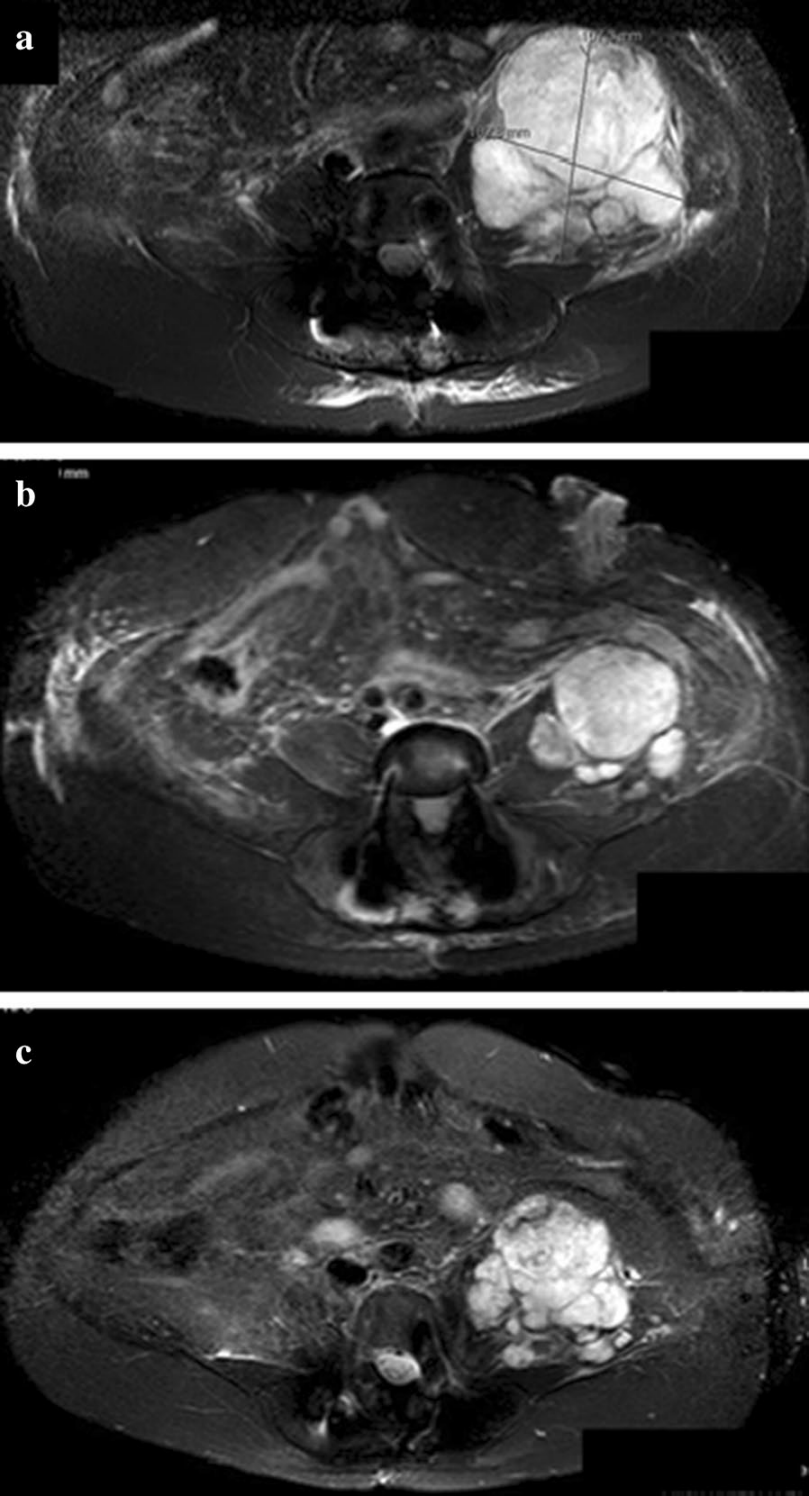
MRI images (T2 gadolinium and fat suppression) of patient 5 treated with sunitinib. a MRI scan at start of sunitinib showing a large retroperitoneal metastasis of a primary lumbar spine chordoma. Measurements: 10.3 × 10.7 × 9.4 cm (LR × AP × CC). b MRI scan after 6 months of sunitinib showing a partial response according to RECIST 1.1. Measurements: 9.5 × 7.4 × 8.3 cm (LR × AP × CC). c MRI scan showing progressive disease after 27 months of treatment. Measurements: 8.9 × 10 cm (LR × AP)
